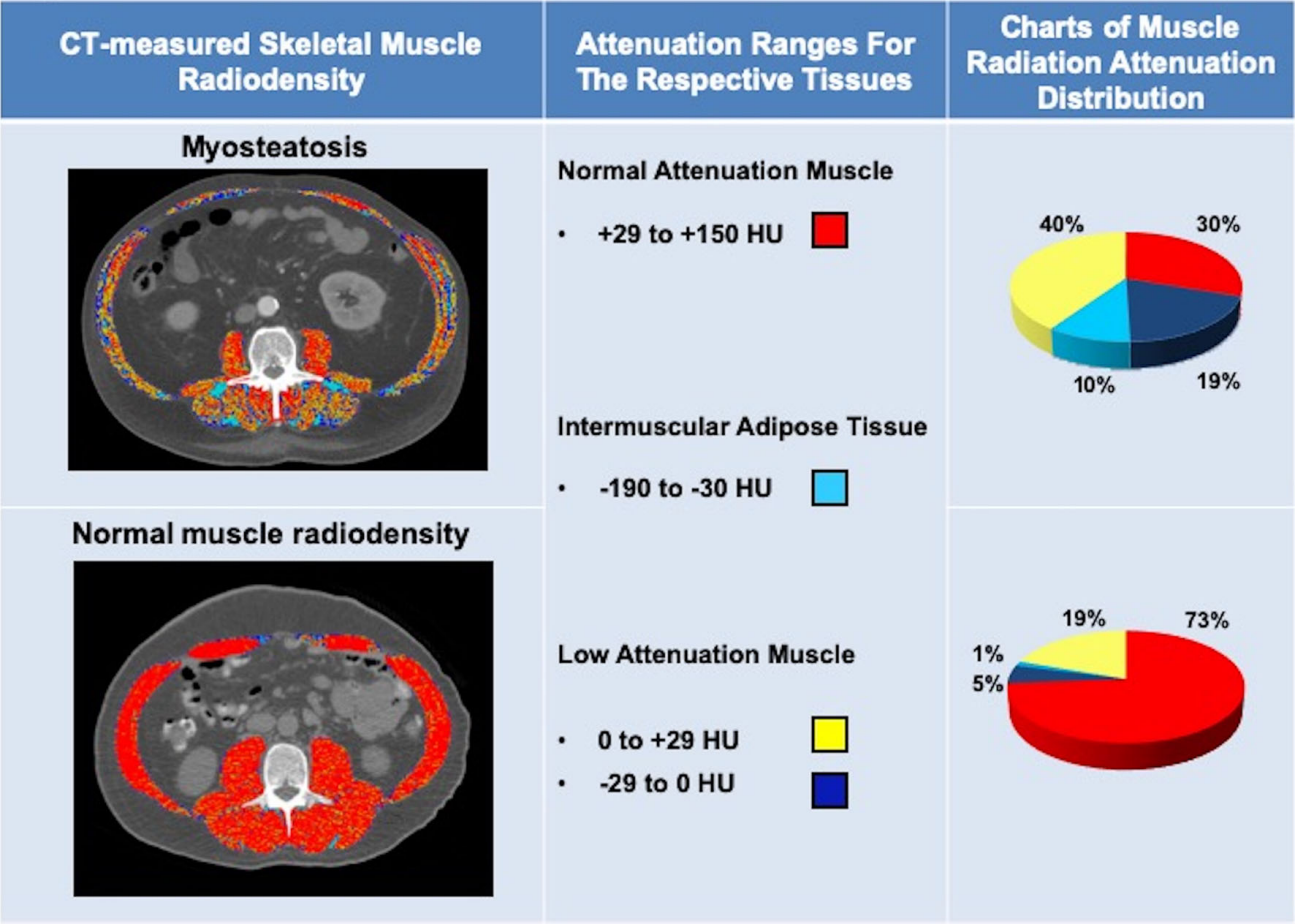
Computed tomography images used for the muscle radiodensity assessment in patients with cirrhosis. Comparison of two patients with cirrhosis and similar BMI. Attenuation ranges used for the analysis of normal attenuation (red), low attenuation region 1 (yellow), and low attenuation region 2 (dark blue) muscle, as well as intermuscular adipose tissue (IMAT; teal), are shown. In patient with low muscle attenuation (34 HU) or myosteatosis, less than half of the muscle cross-sectional area falls within the normal attenuation range whereas in patient with normal muscle attenuation (47 HU, no-myosteatosis), muscle with the normal attenuation range is predominant
